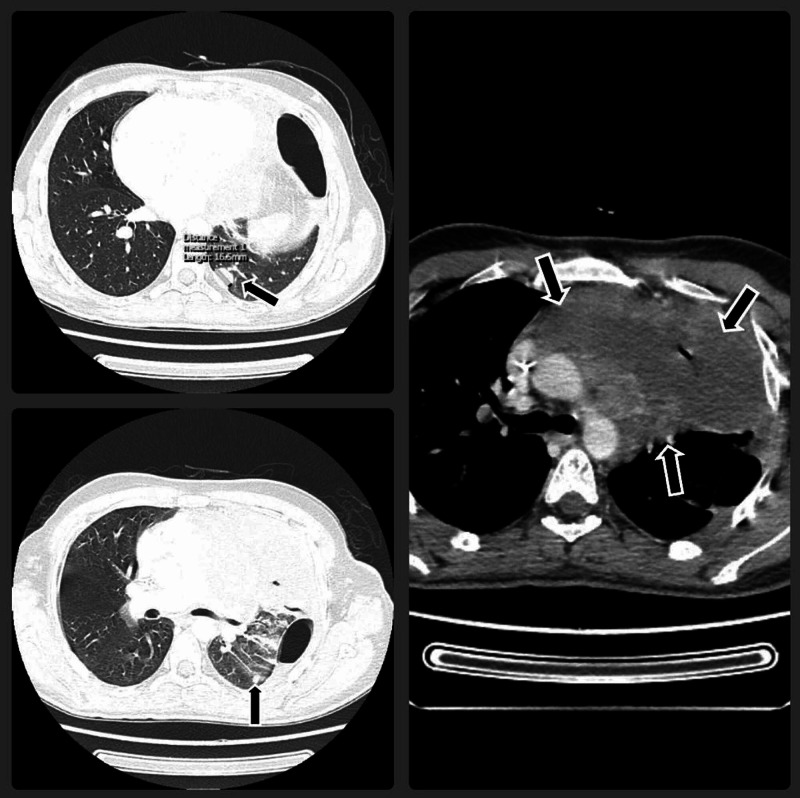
Computed tomography (CT) of the chest following chemotherapy shows residual mass in the superior mediastinum with appearance of two new lung lesions.
